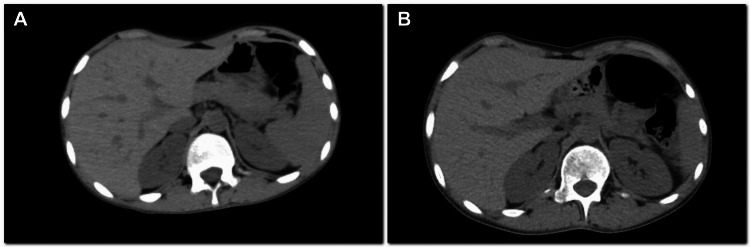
Noncontrast CT chest axial images. (A) Acquired one week after previous MRI and (B) acquired at the time of end of treatment, six months later. Partially covered liver shows no lesions
